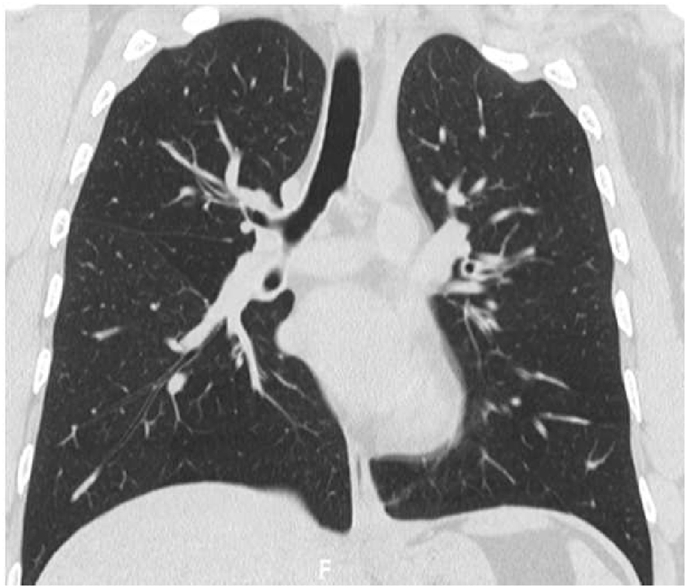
March 2017, CT chest, lung window, showing bilateral bronchial wall thickening and atelectatic bands in the right upper lobe.
